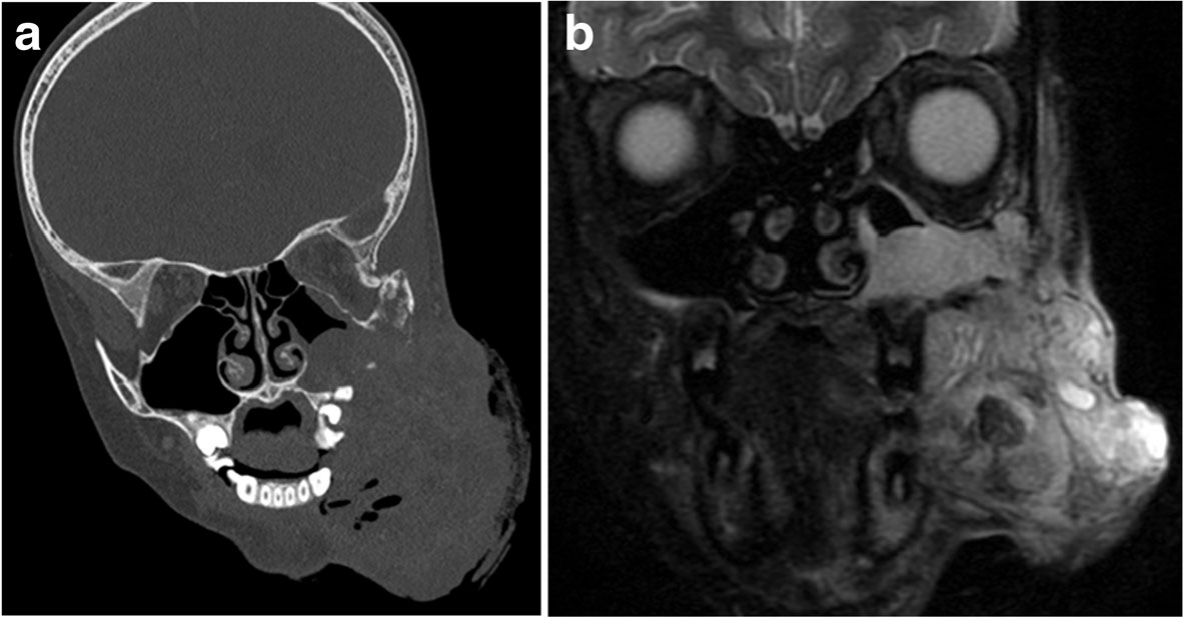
Biphenotypic sinonasal sarcoma. Coronal CT (a) and fat-suppressed T2-weighted MRI (b) show an aggressive bulky mass involving the left maxillary sinus and lower face
